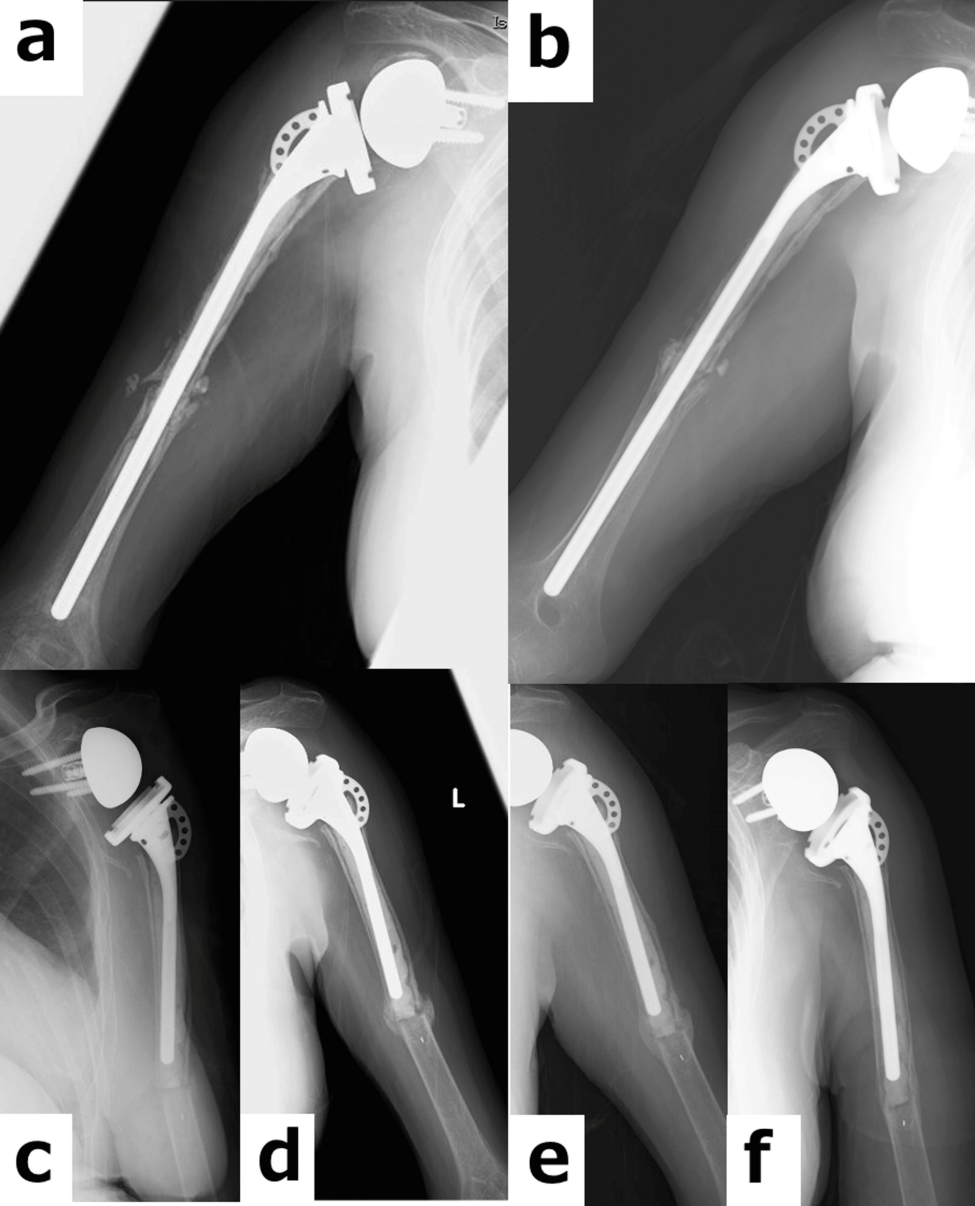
Radiographic follow-up at both shoulders (a) Radiograph at six months after revision surgery. (b) Radiograph at 15 months after revision surgery. (c) Radiograph at one month after re-injury. (d) Radiograph at three months after re-injury. (e) Radiograph at six months after re-injury. (f) Radiograph at 12 months after re-injury.
